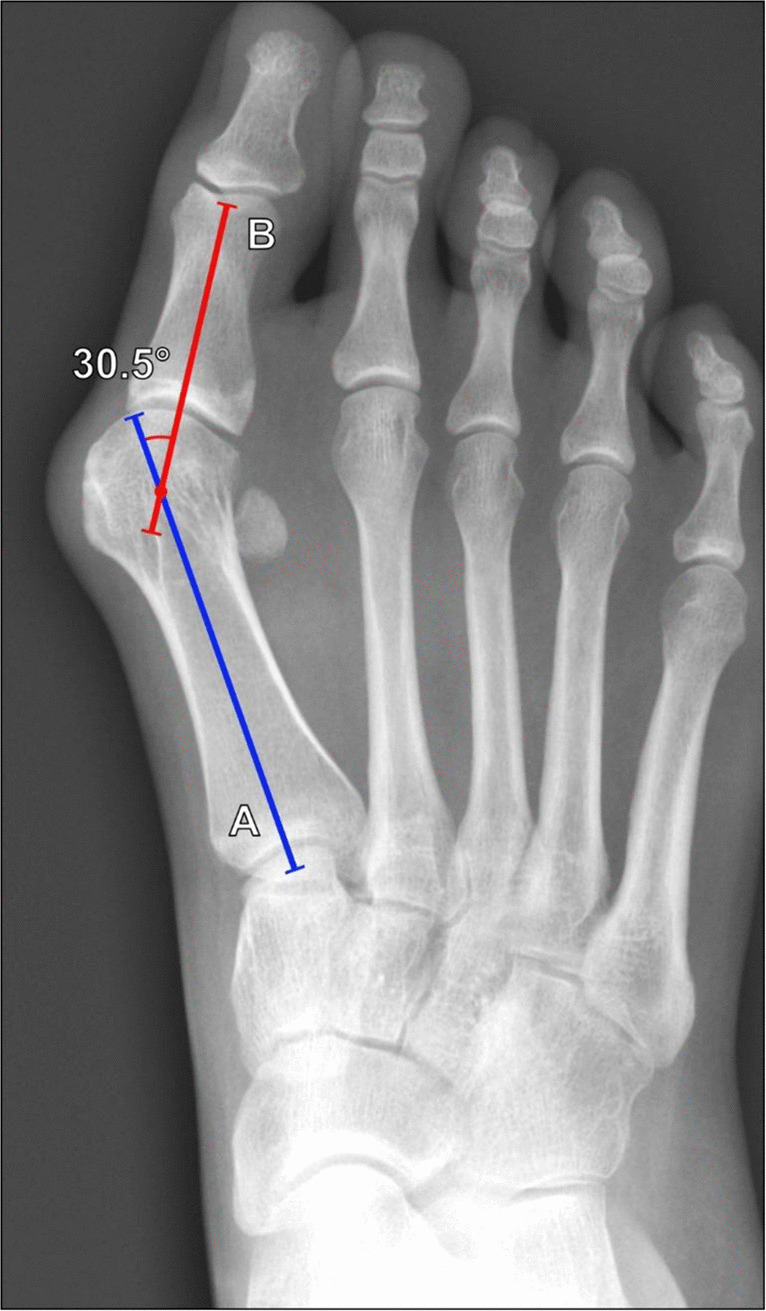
Measurement of hallux valgus (first metatarsophalangeal) angle on DP radiograph of the foot in a 32-year-old female patient with forefoot pain and deformity. The angle is formed between the long axis of the first metatarsal (A, blue line) and the first proximal phalanx (B, red line). The normal angle measures < 15°, and an angle ≥ 15° reflects hallux valgus. Radiographically, there is an increased first metatarsophalangeal angle, indicating moderate hallux valgus, with bunion formation and lateral hallux sesamoid rotation
